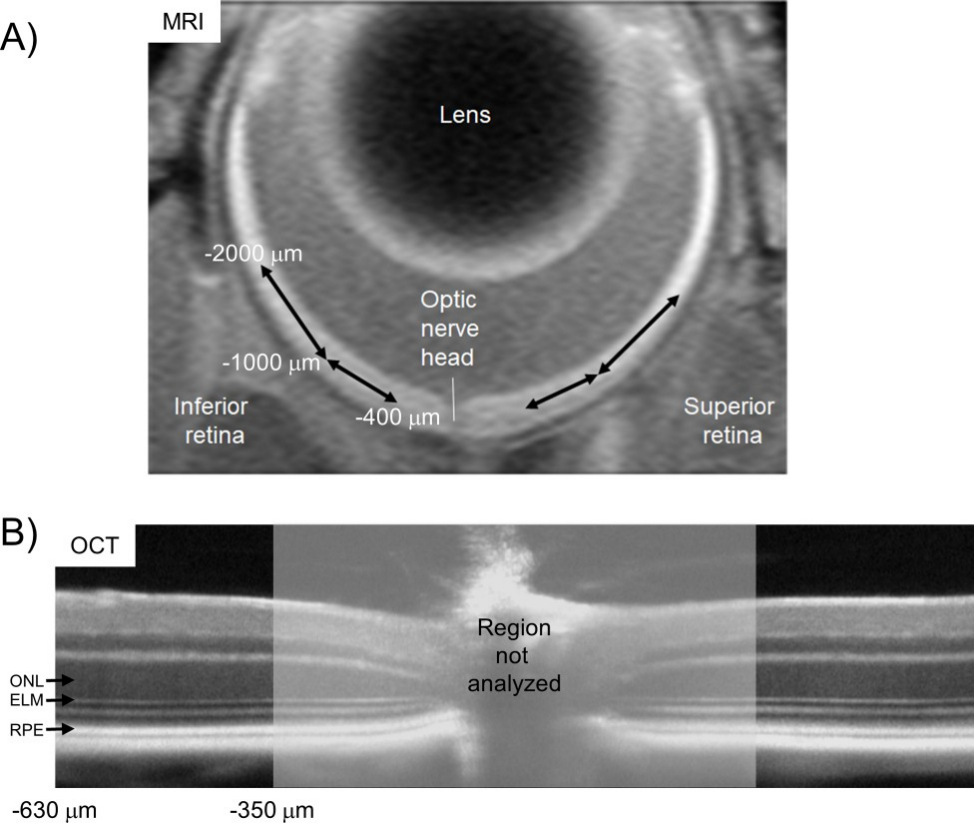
Comparison of MRI and OCT regions-of-interest (ROI’s). Four non-overlapping retinal regions were evaluated from MRI data A): ± 400–1000 μm and ± 1000–2000 μm from the optic nerve head for their transretinal 1/T1 profiles (shown in Figs 3 and 6). Two regions in central retina were studied from OCT data B): ± 350–630 μm from the optic nerve head to determine outer nuclear layer (ONL) thickness, and the external limiting membrane–retinal pigment epithelium (ELM-RPE) thickness.
